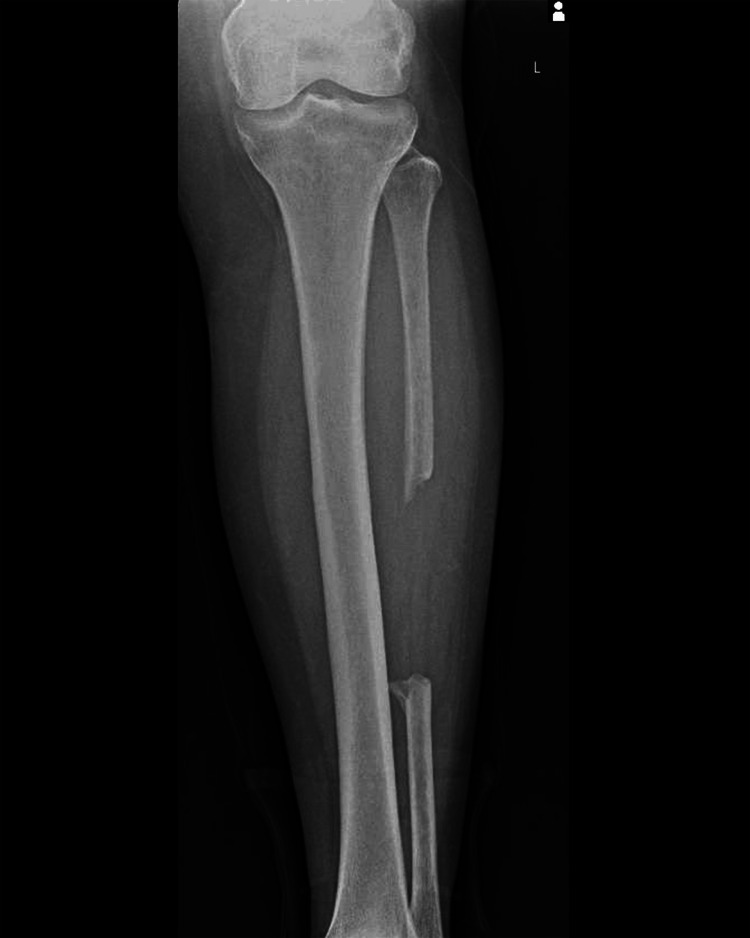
Anteroposterior X-ray of the left leg taken two years postoperatively, with visible fibula shaft graft harvest site shown
